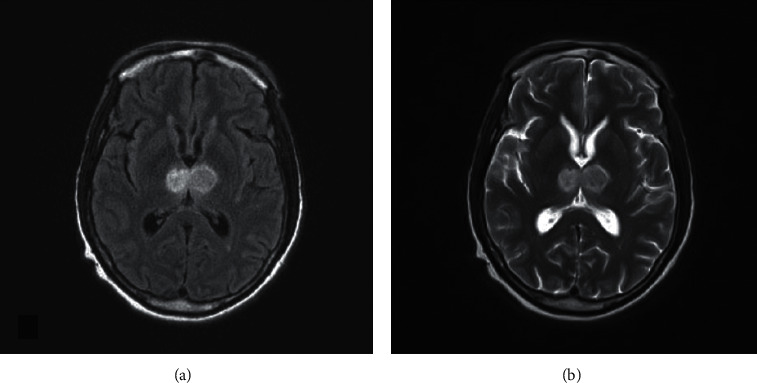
Axial flair (a) and axial T2 (b) MR images obtained one week after the CT demonstrated increased signal bilaterally in the thalamic nuclei.
